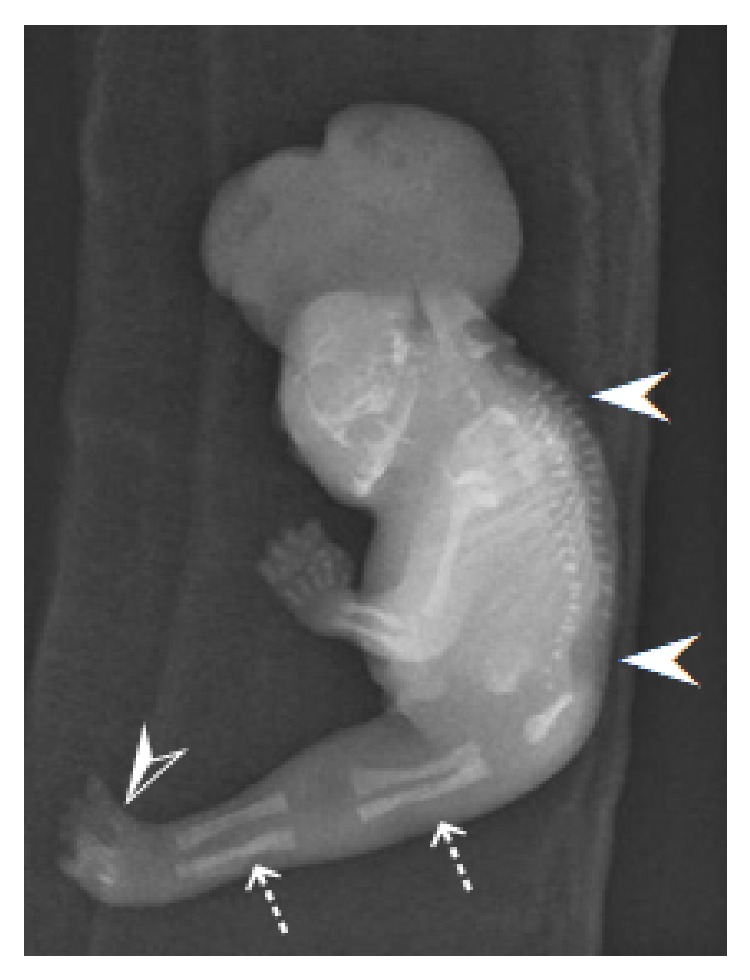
Autopsy imaging by radiography. The radiographs demonstrated complete rachischisis (white arrowheads) and the single lower limb containing two femora and two tibiae (white dashed arrows) and some metatarsals and phalanges (white open arrowhead).
